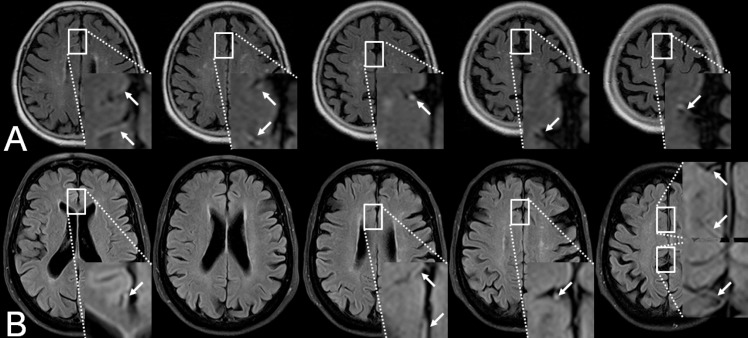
Two examples of FVH in the distal branches of the anterior cerebral artery (ACA, arrows) on FLAIR images in acute ischemic stroke due to ACA occlusion.
